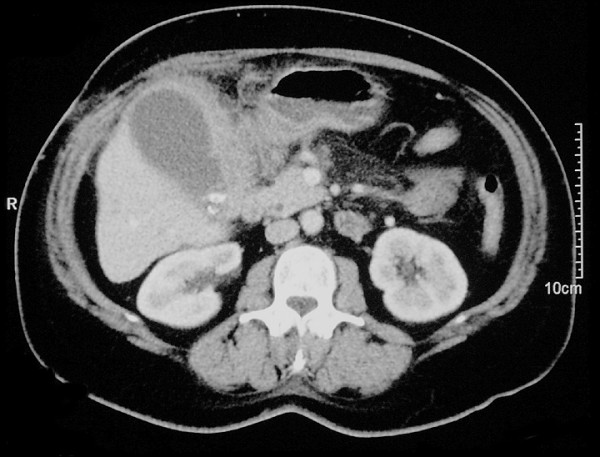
CT scan showing the gallbladder inflammatory mass
